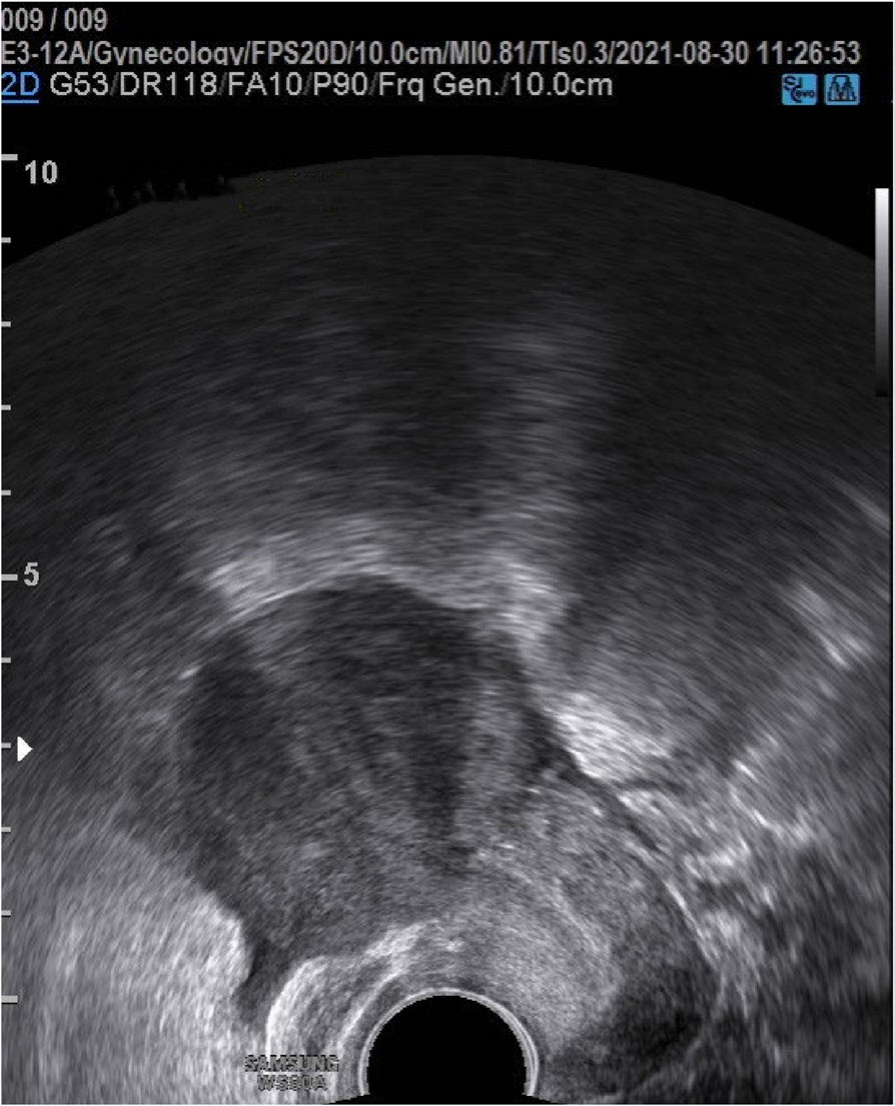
The ultrasound examination at one month after surgery showed the myometrium of the lower uterine segment was continuous
